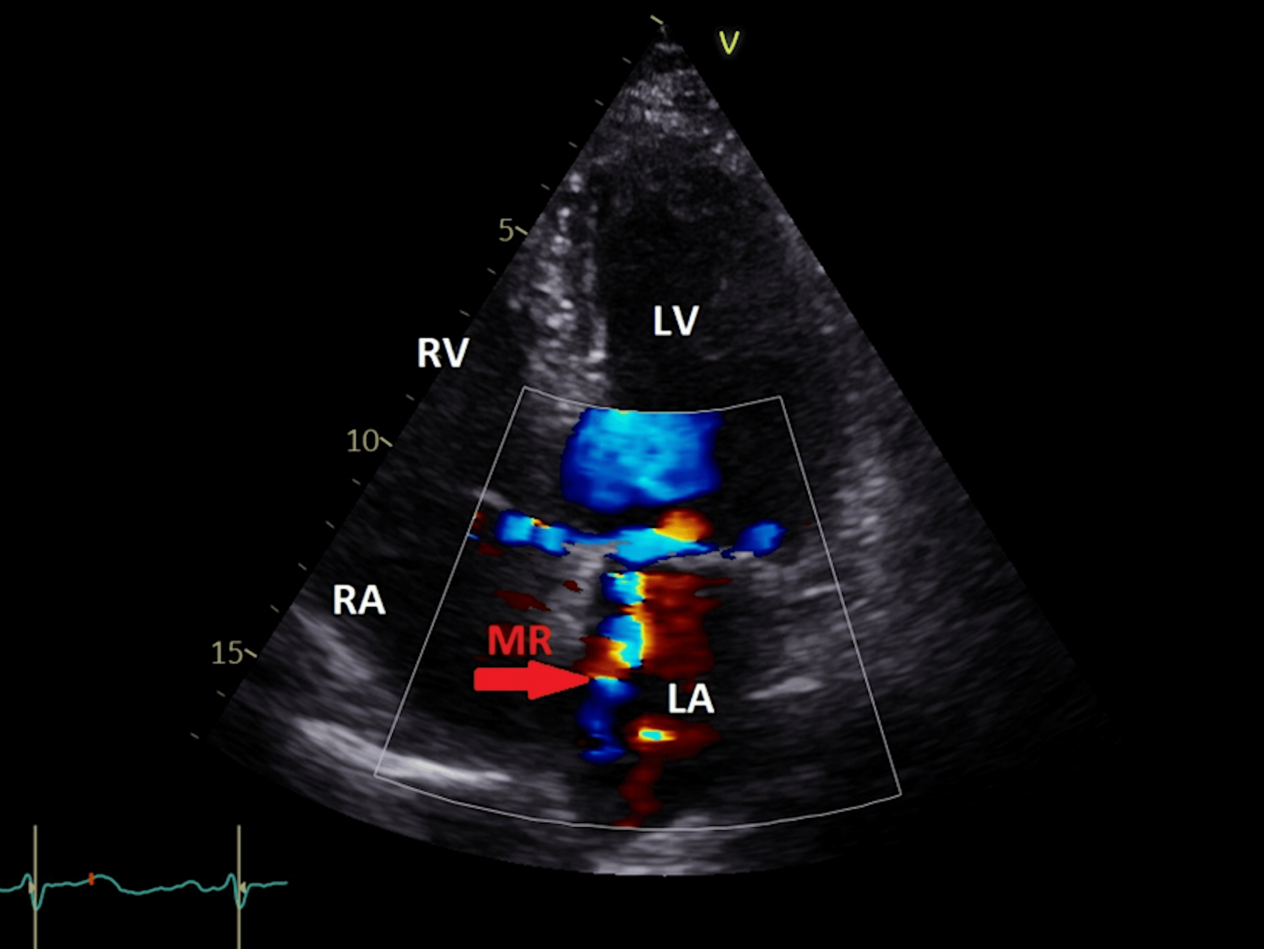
Apical four-chamber view showing grade II mitral regurgitation by color flow (red arrow). LA = left atrium; LV = left ventricle; RA = right atrium; RV = right ventricle
